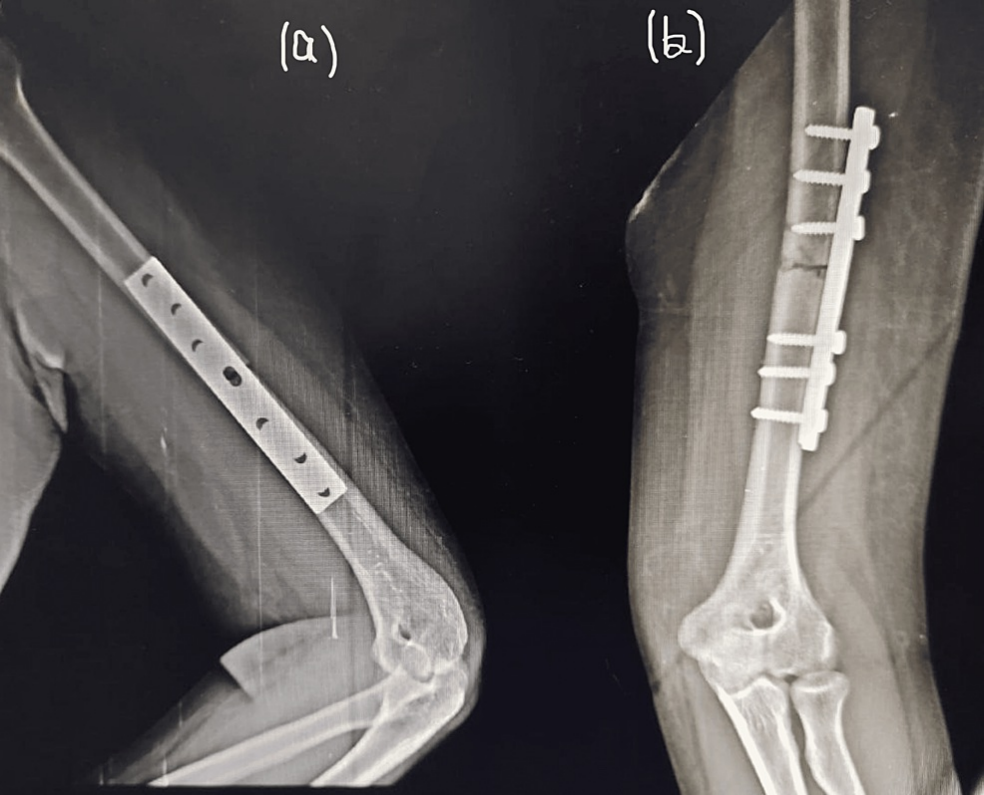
Radiograph showing fracture site six weeks after the internal fixation Left humerus and elbow joint (a) lateral view, (b) anteroposterior view
